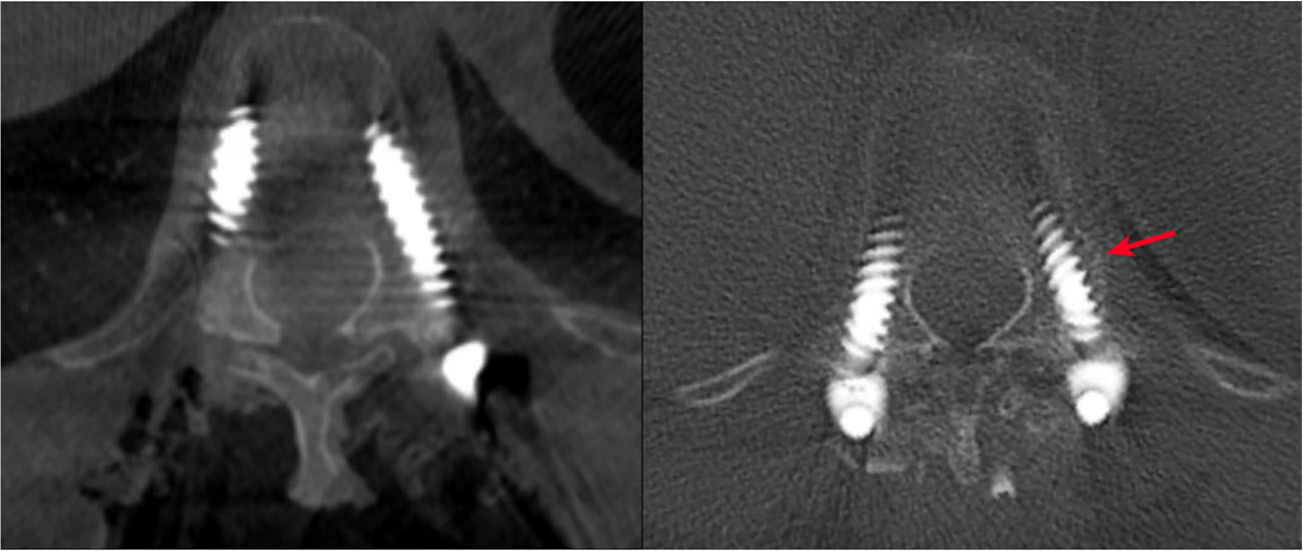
Comparison between CBCT (left) and CT images (right) depicting suspected bone overgrowth between the two images were taken. Red arrow highlights suspected bone overgrowth
